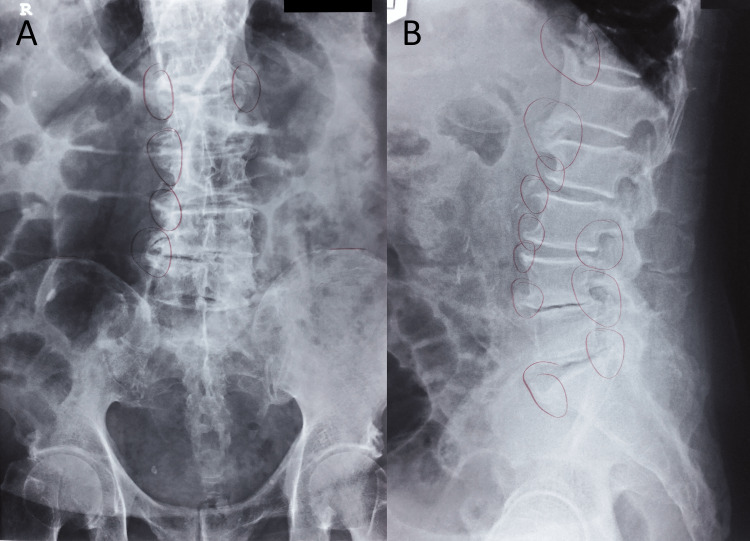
Lumbar radiographs: anteroposterior (A) and lateral (B) views. These radiographs were taken at the patient’s orthopedic re-evaluation in 2020, soon before he presented to the chiropractor, and were reported to reveal worsening of the lumbar spondylosis. The patient’s orthopedist drew markings (circles) on the film indicating areas of spondylosis.
